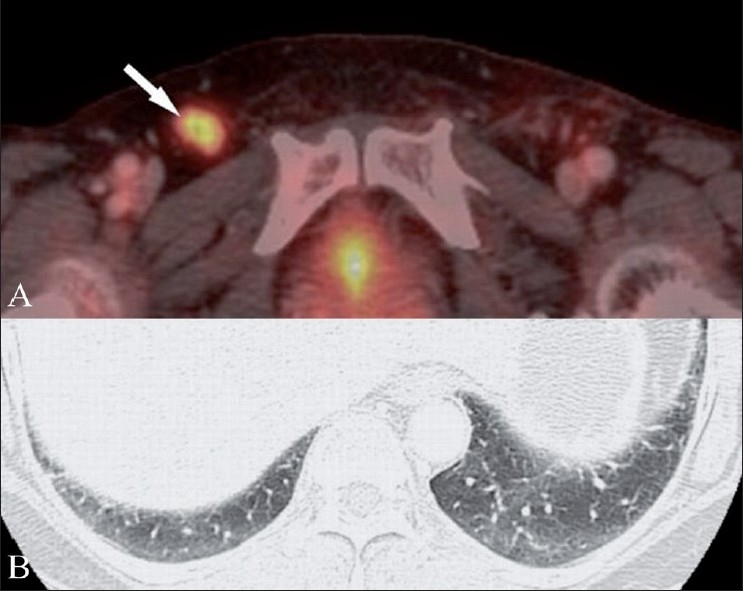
Follow-up FDG PET/CT (A) after penicillin therapy shows decreased FDG uptake in the right inguinal lymph node (arrow). Follow-up CT scan of the chest (B) obtained 2 months after discharge shows complete disappearance of the pulmonary nodules
